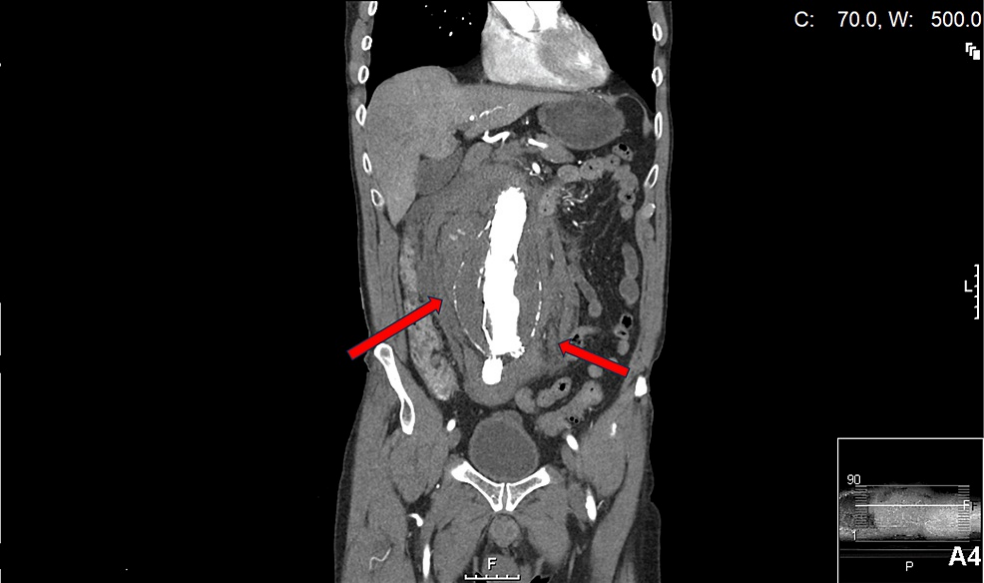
CT abdomen and pelvis coronal view showing AAA with hypo- and hyperdense-blood products spilling into the abdomen and pelvis CT: computed tomography; AAA: abdominal aortic aneurysm
